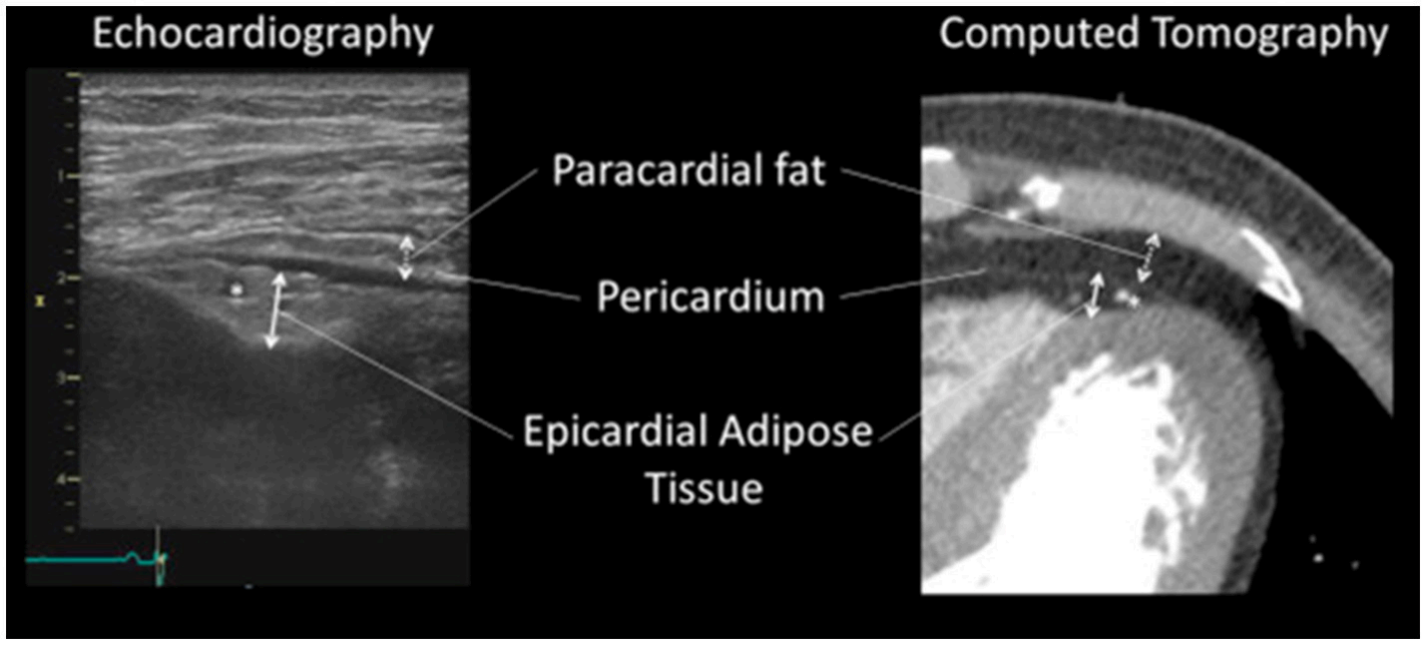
Identification of epicardial adipose tissue and paracardial adipose tissue. Identification of epicardial adipose tissue and paracardial adipose tissue by echocardiography (Left) and contrast enhanced 320-slice multi-detector computed tomography (Right). The asterisks (^*^) indicate left descending coronary artery. “Pericardial adipose tissue” includes both epicardial adipose tissue (located within or deep into the pericardium) and paracardial adipose tissue (located superficial to the pericardium). Sometimes, pericardial adipose tissue is referred to as paracardial adipose tissue. All figures cited from the reference (Yamada and Sata, 2015) without modification.
